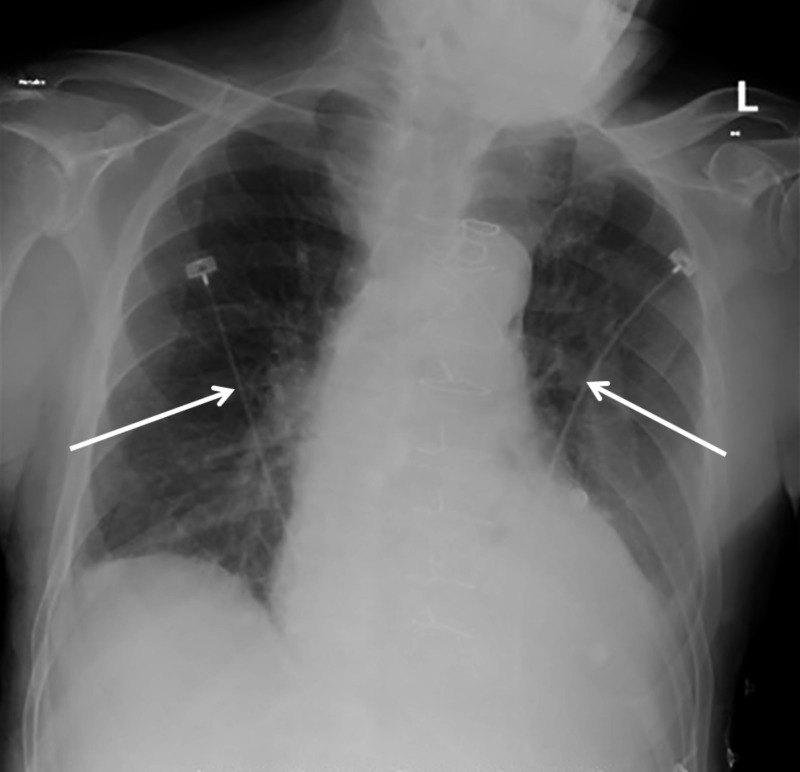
Chest radiography demonstrating pulmonary venous congestion.
